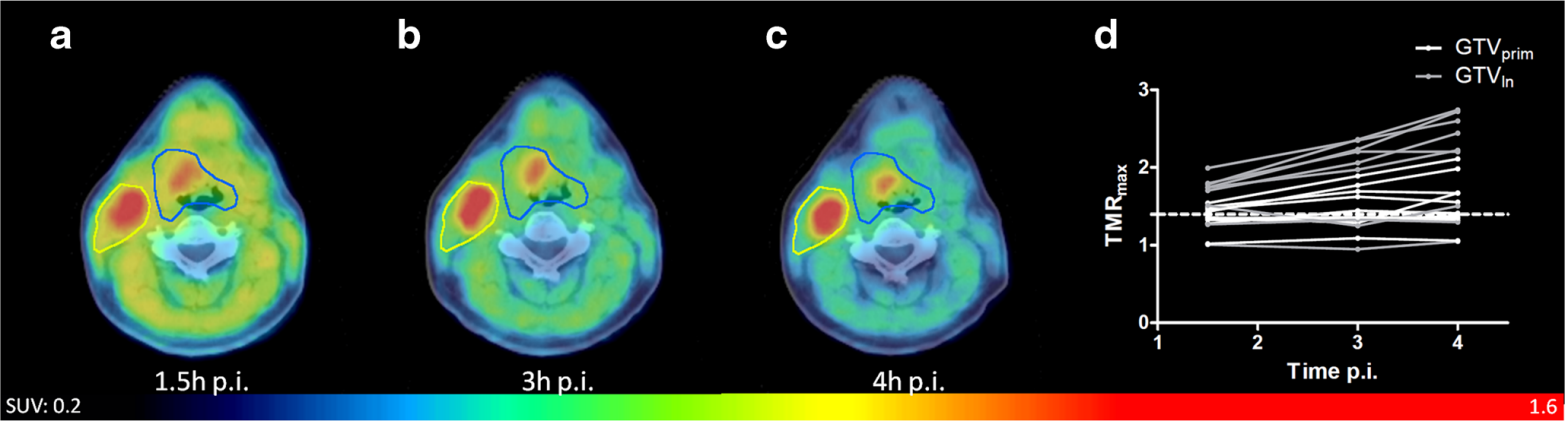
[^18^F]HX4 PET/CT scans of a patient with a T2N2bMx squamous cell carcinoma of the oropharynx, scanned at 1.5 h (*a*), 3 h (b), and 4 h p.i. (c). d: The tumour to muscle ratio (TMR_max_) for all patients. Shown are the gross tumour volumes of the primary lesions (GTV_prim_) and the metastatic lymph nodes (GTV_ln_), which increased significantly (1.5 h vs 3 h: *p* < 0.01, 3 h vs 4 h: *p* = 0.02)
